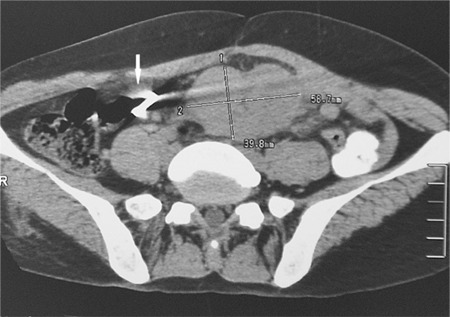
Abdominal CT reveals a mass of 5.9 x 4.0 cm in size adjacent to the aorta abdominalis under the umbilicus, to the right of which a dissociative circinate foreign body of 2 cm in diameter is observed (arrow).
